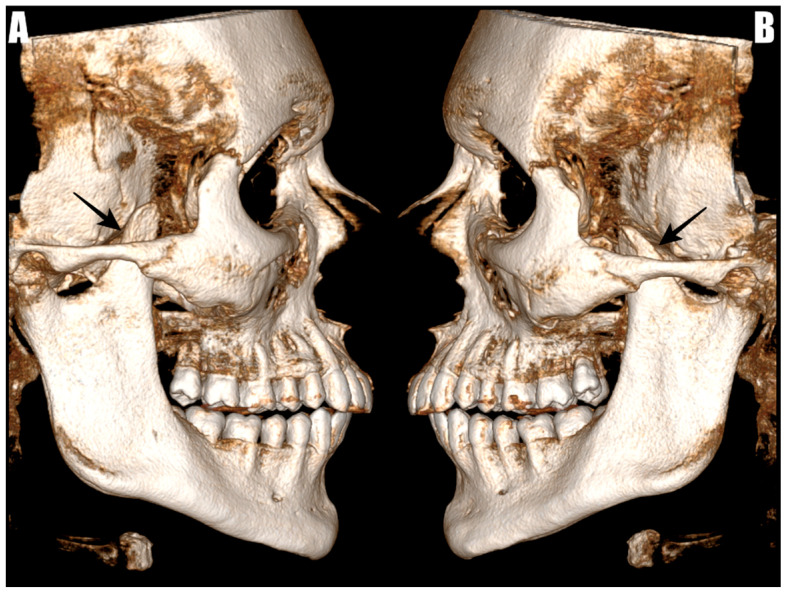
The 3D reconstruction of a CBCT image showing elongated coronoid processes indicated by arrows. (A) Represents the right side and (B) represents the left side.
